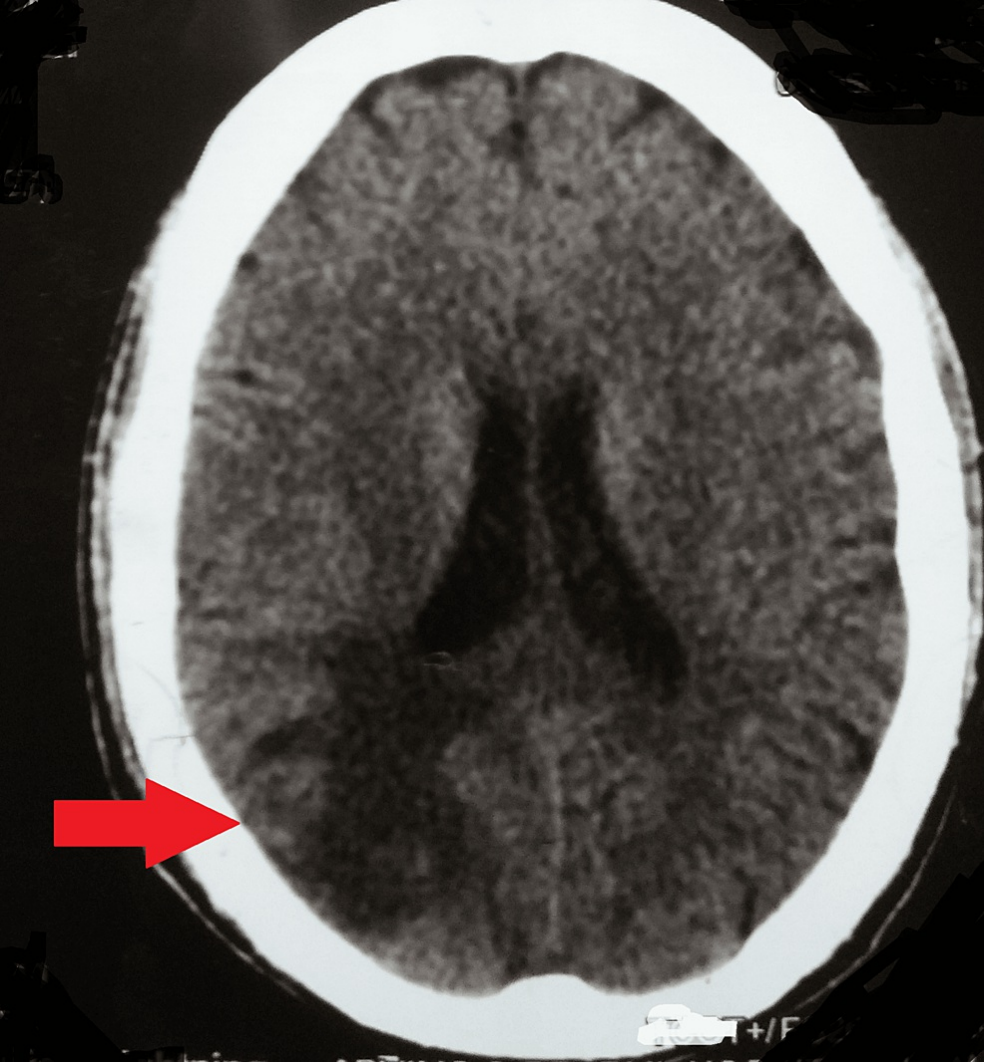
An MRI of the brain showed involvement of the right cerebral hemisphere with border zone infarct MRI: Magnetic resonance imaging
